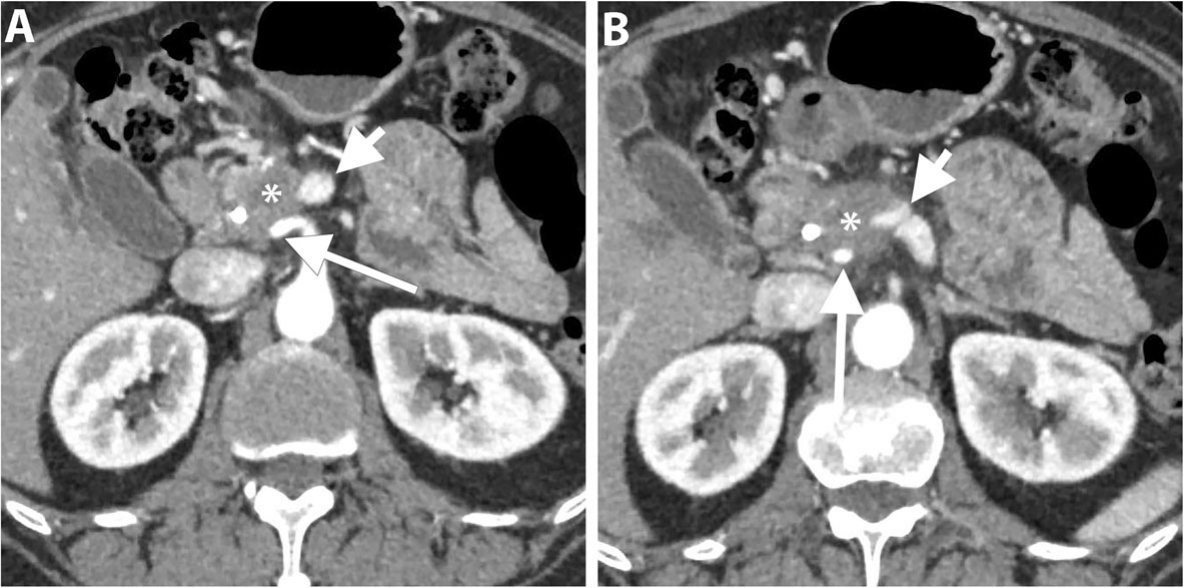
A 63-year-old man with PDAC. Axial pancreatic phase CT images (a and b) show a hypoattenuating mass (asterisk) in the pancreatic head with > 180° tumor contact with a replaced right hepatic artery (long arrow) and > 180° tumor contact with SMV (short arrow) with deformity of the vein lumen. The patient underwent a total pancreatectomy and vascular reconstruction after neoadjuvant therapy, but liver metastases developed one year after surgery
